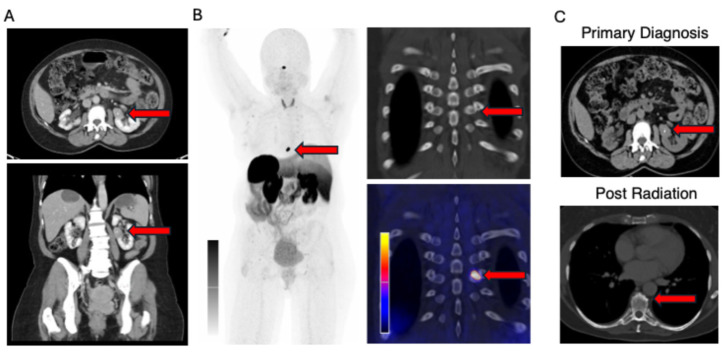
Radiological findings. (A) Initial preoperative CT scan showing a left-sided renal tumor (red arrows). (B) Postoperative SSTR PET/CT revealing a suspected metastatic lesion in thoracic vertebra 8 (T8) with high SSTR expression (red arrows). (C) Additional CT scan showing the left-sided renal tumor at first diagnosis (red arrows) and metastatic lesion at thoracic vertebra 8 (T8) post radiation (red arrow). Abbreviations: CT = Computed Tomography, SSTR PET/CT = Somatostatin Receptor Positron Emission Tomography/Computed Tomography.
